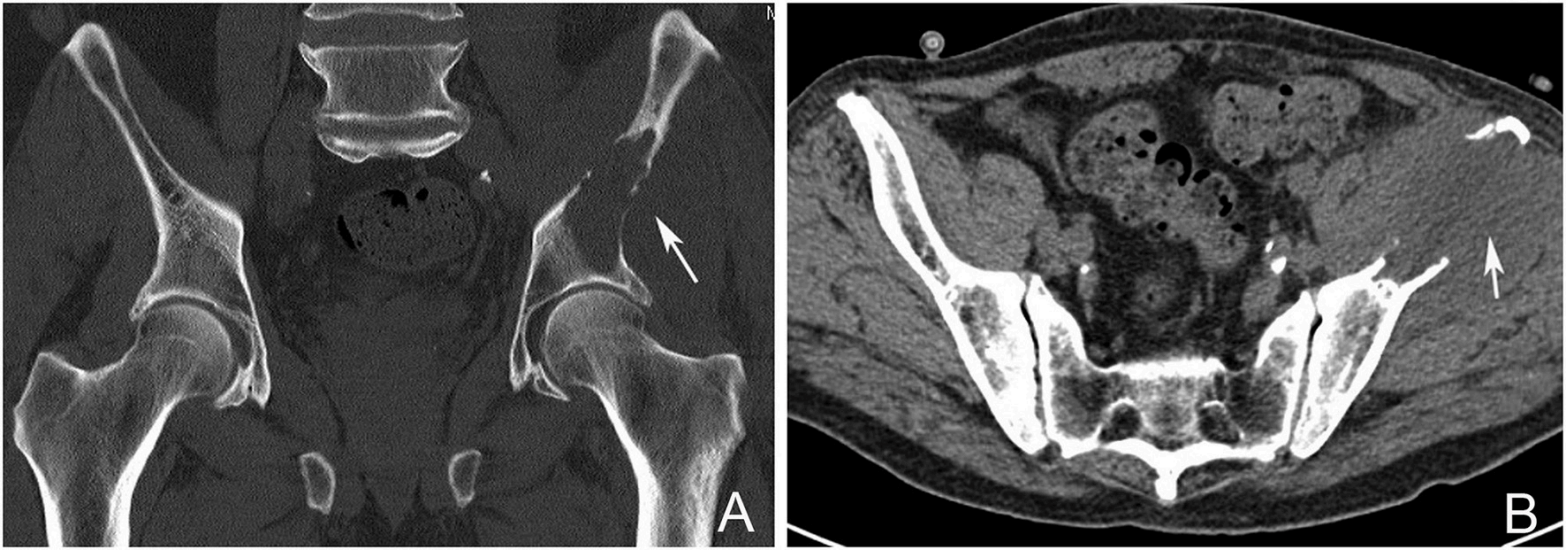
Pelvic CT Scan showing a left sided periacetabular renal cell carcinoma metastasis. (A) Involvement of the left supraacetabular region by a large lytic metastatic lesion (arrow). (B) Note the extensive extraosseous involvement (arrow).
